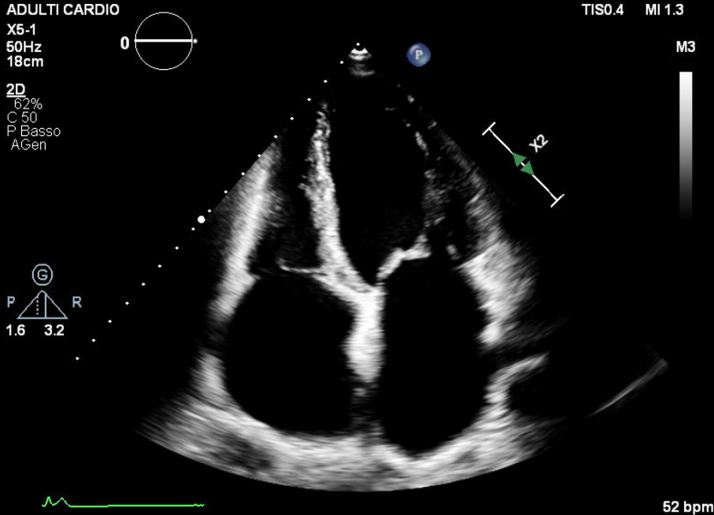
2-Dimensional Transthoracic Echocardiography, Apical 4-Chamber View of the Proband, Showing Marked Biatrial Enlargement With Preserved Biventricular Size and Systolic Function
